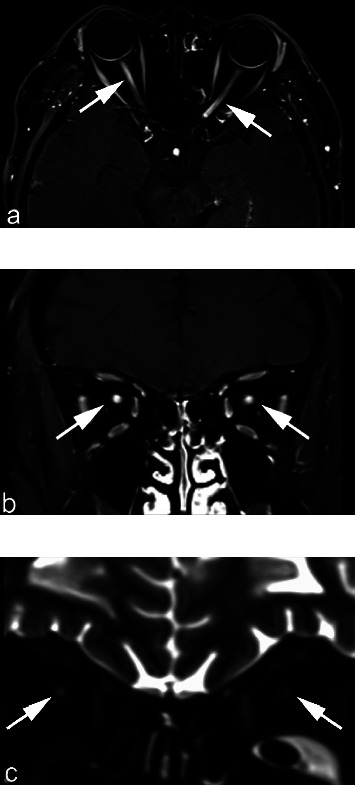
MRI identified optic nerve lesions in patients with methanol-induced optic neuropathy. Axial (a) and coronal (b) T1 enhancement images of patient 4, demonstrating hyperintense signals (arrows) in both optic nerves; (c) coronal T2 image of patient 8, demonstrating hyperintense signals (arrows) in both optic nerves.
